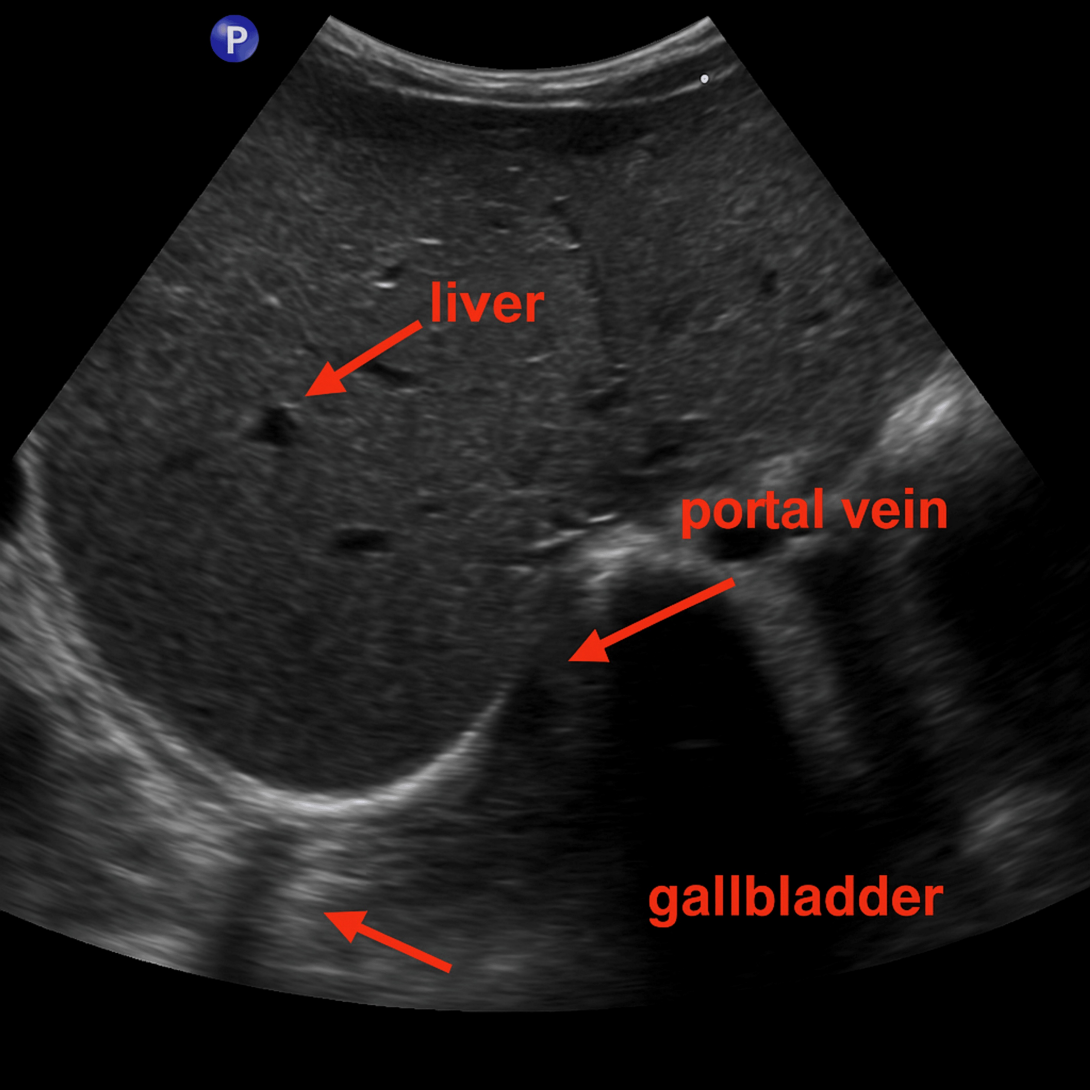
Ultrasonography image of case 3
